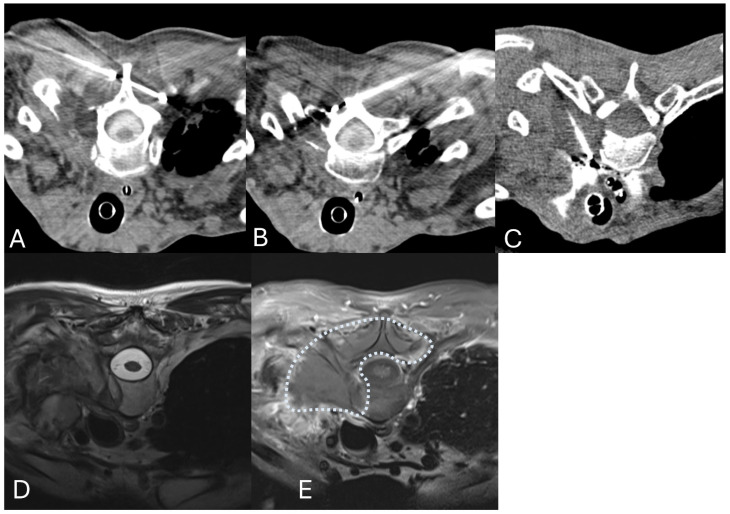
Cryoablation allows shapable thermal margins: (A–C) Variable-sized cryoablation probes placed under CT guidance over the lamina of T1 and adjacent to the spine at T1/2 on the right with thermal ice ball (hypointense region around the probes) at 8 min. (D,E) Axial FSE T2WI and Axial fat-saturated T1WI after gadolinium allow visualization of the thermal margin 6 months after treatment (dashed line).
